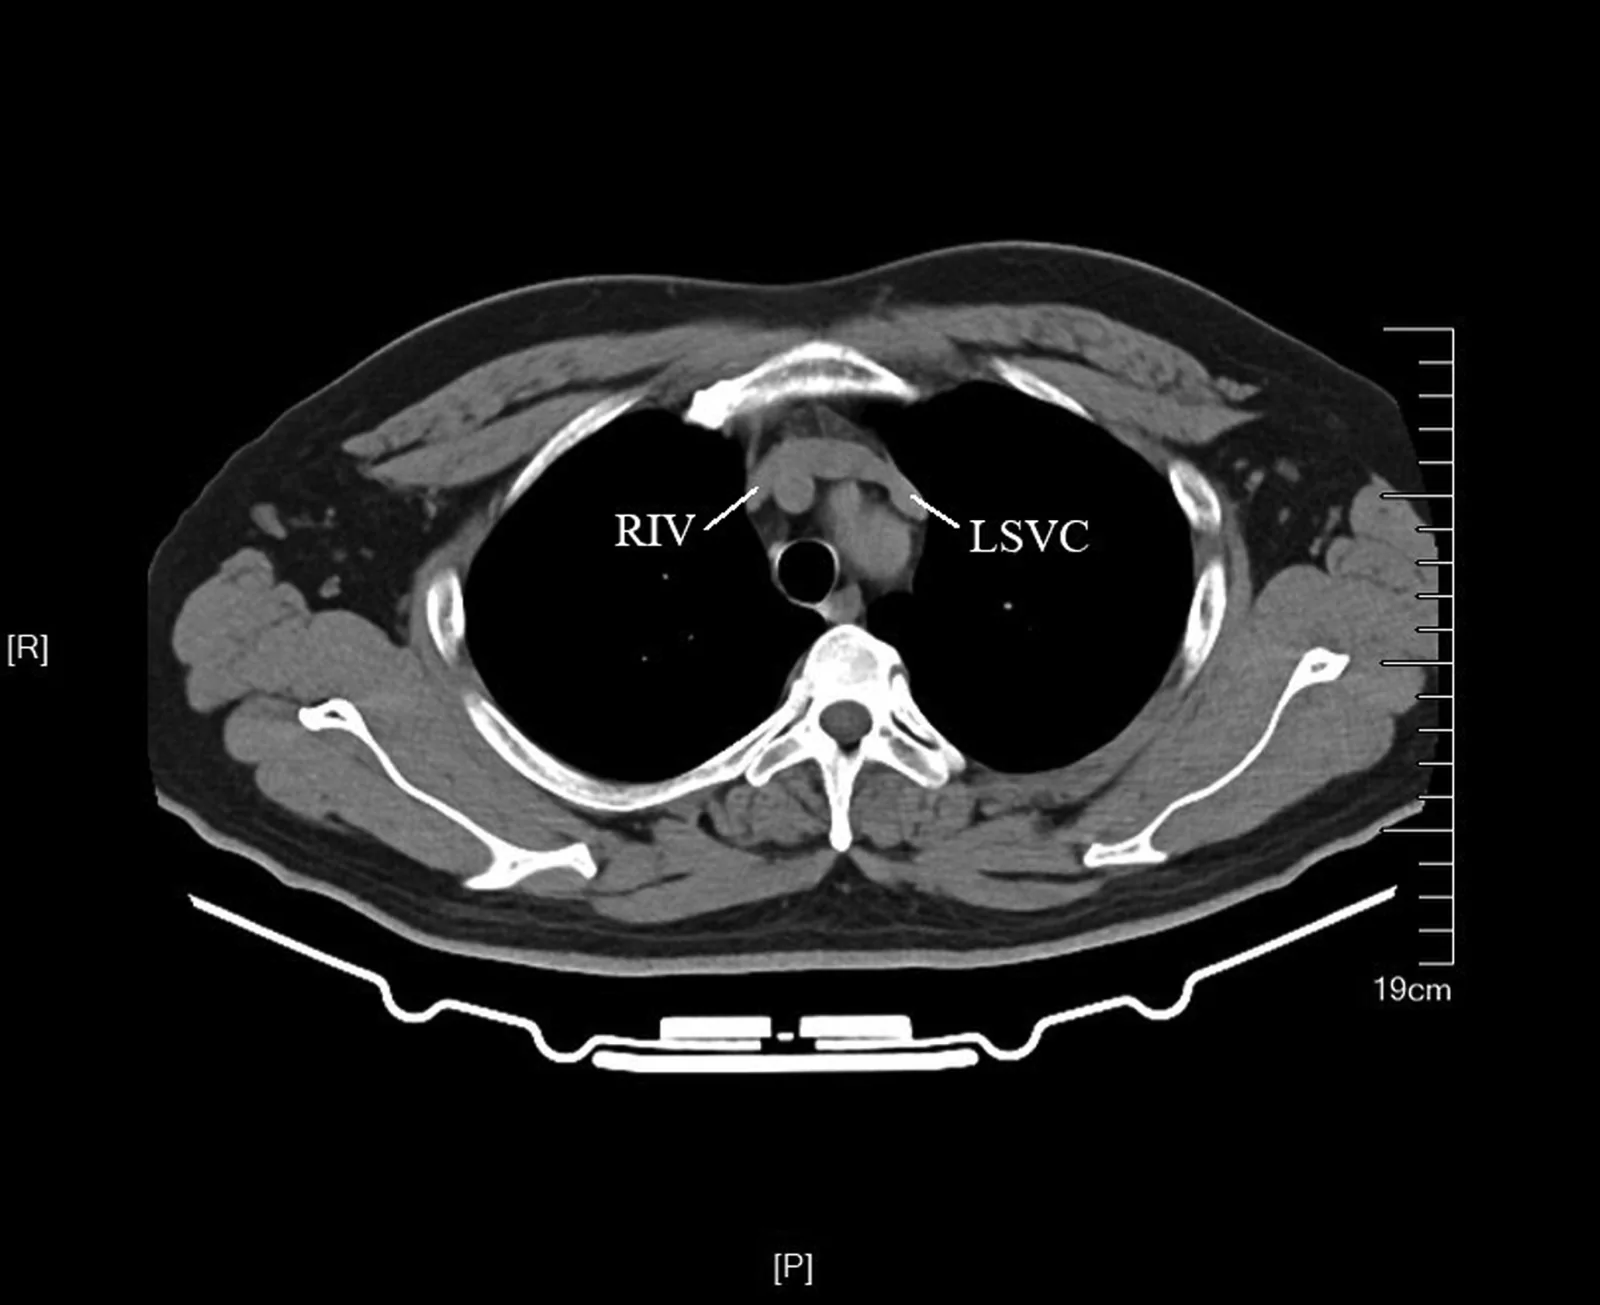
Axial section of CT thorax at (mediastinal window) showing no right superior vena cava, but a right innominate vein merging into the left superior vena cava. CT = computed tomography, LSVC = left superior vena cava, RIV = right innominate vein.
